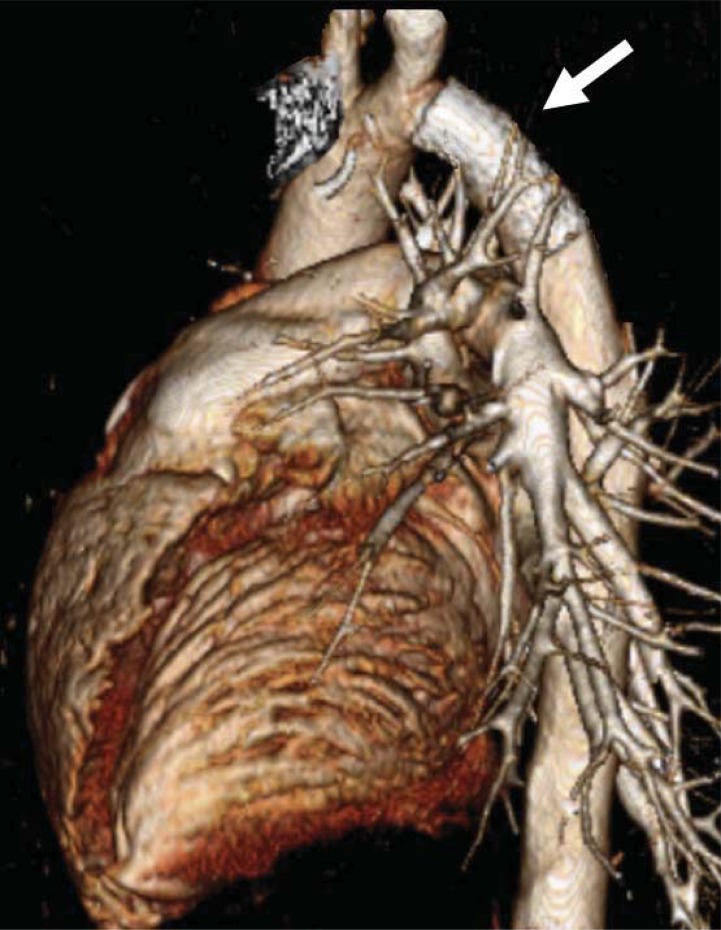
Computed tomography scan with three-dimensional reconstruction
